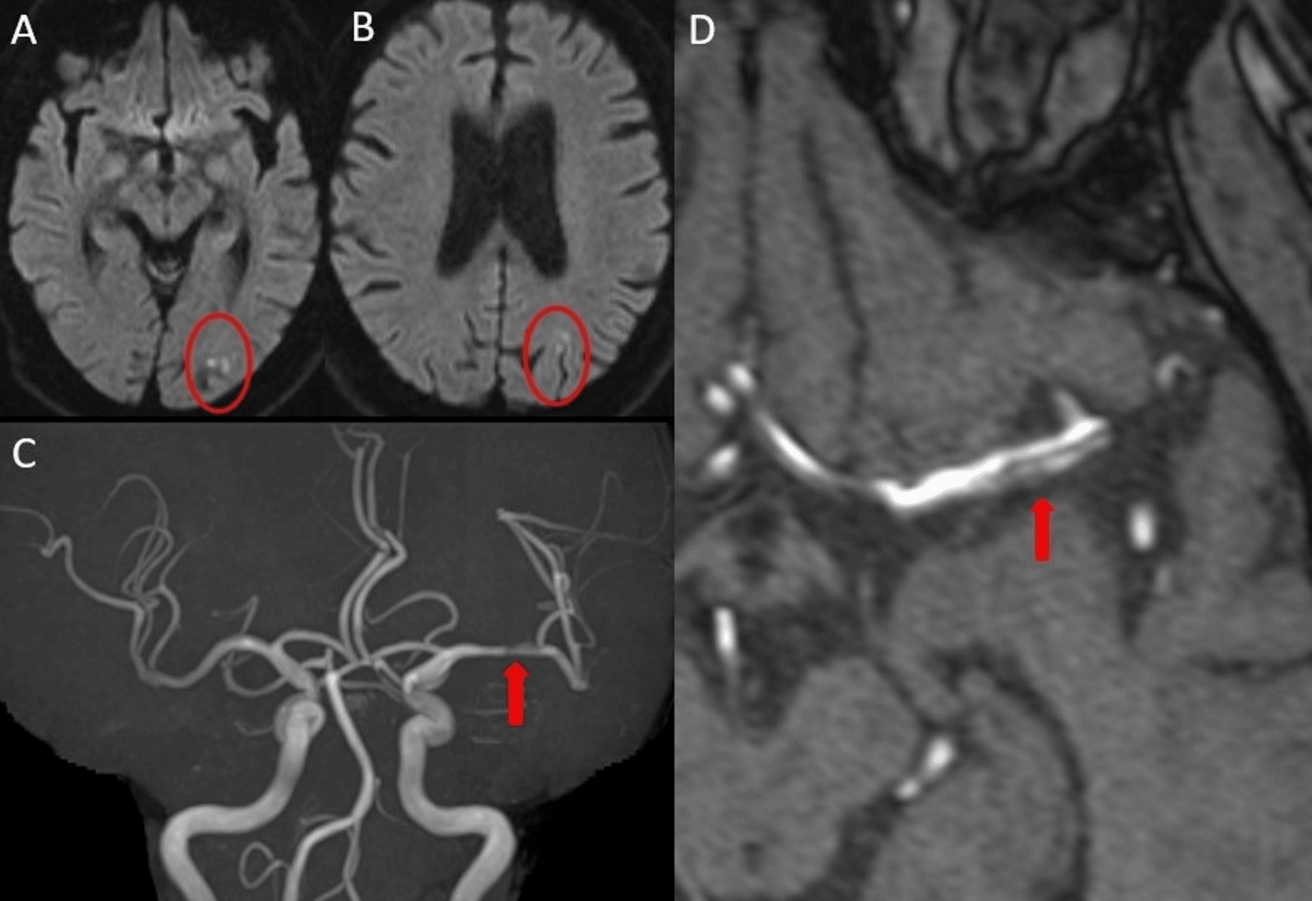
Case 2: Initial imaging on admission (A, B) DWI showing acute ischemic stroke in the left cerebral watershed area (red ovals). (C, D) MRA demonstrating findings suggestive of dissection in the horizontal (M1) segment of the left MCA (arrow). DWI, diffusion-weighted imaging; MCA, middle cerebral artery; MRA, magnetic resonance angiography
